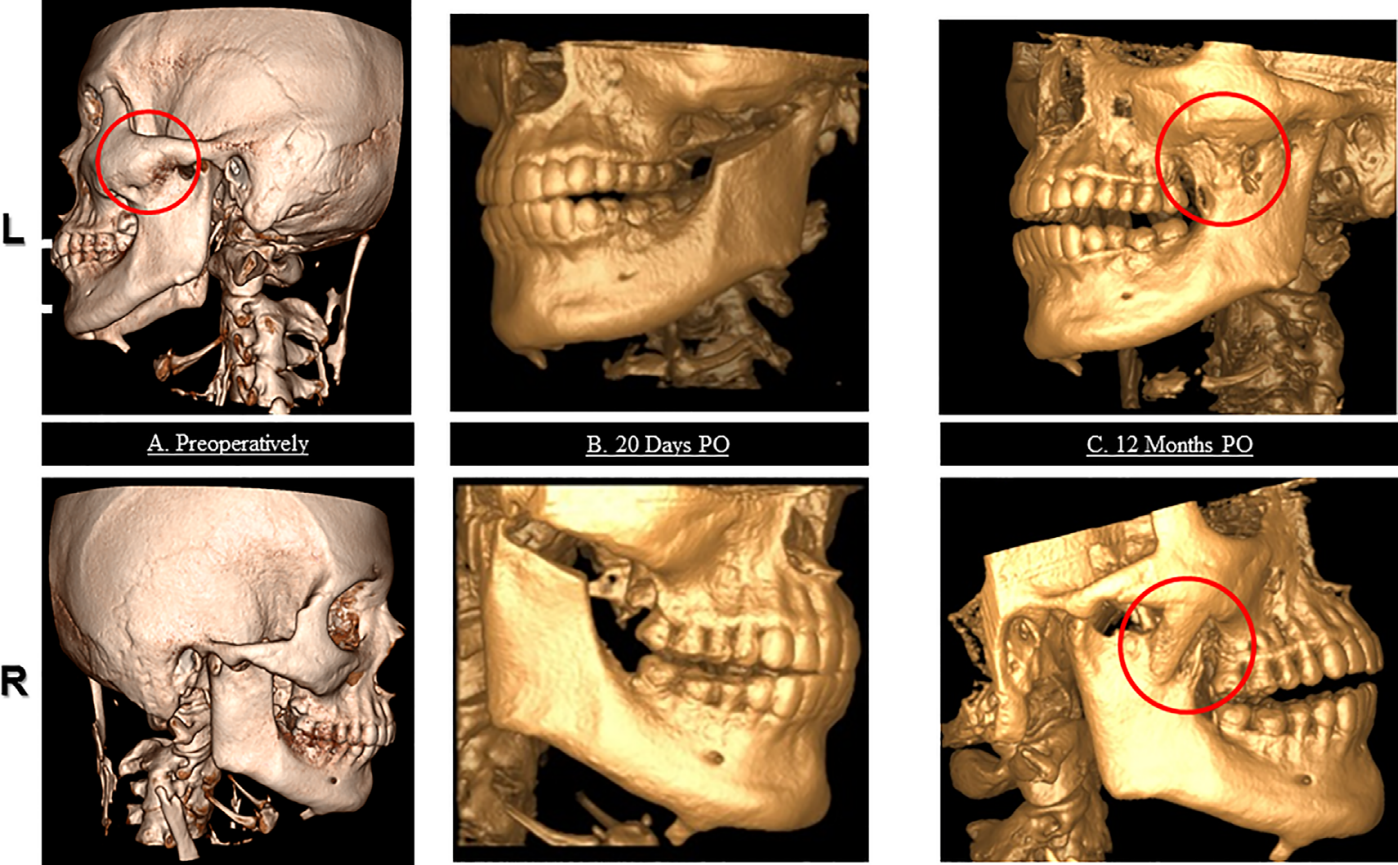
3D‐CT scan reconstruction of the skull in left (upper panel) and right (lower panel) lateral view before bilateral coronoidectomy (*A*), 20 days (*B*), and 12 months (*C*) PO. Heterotopic lesions are marked by red circles. PO = postoperatively.
